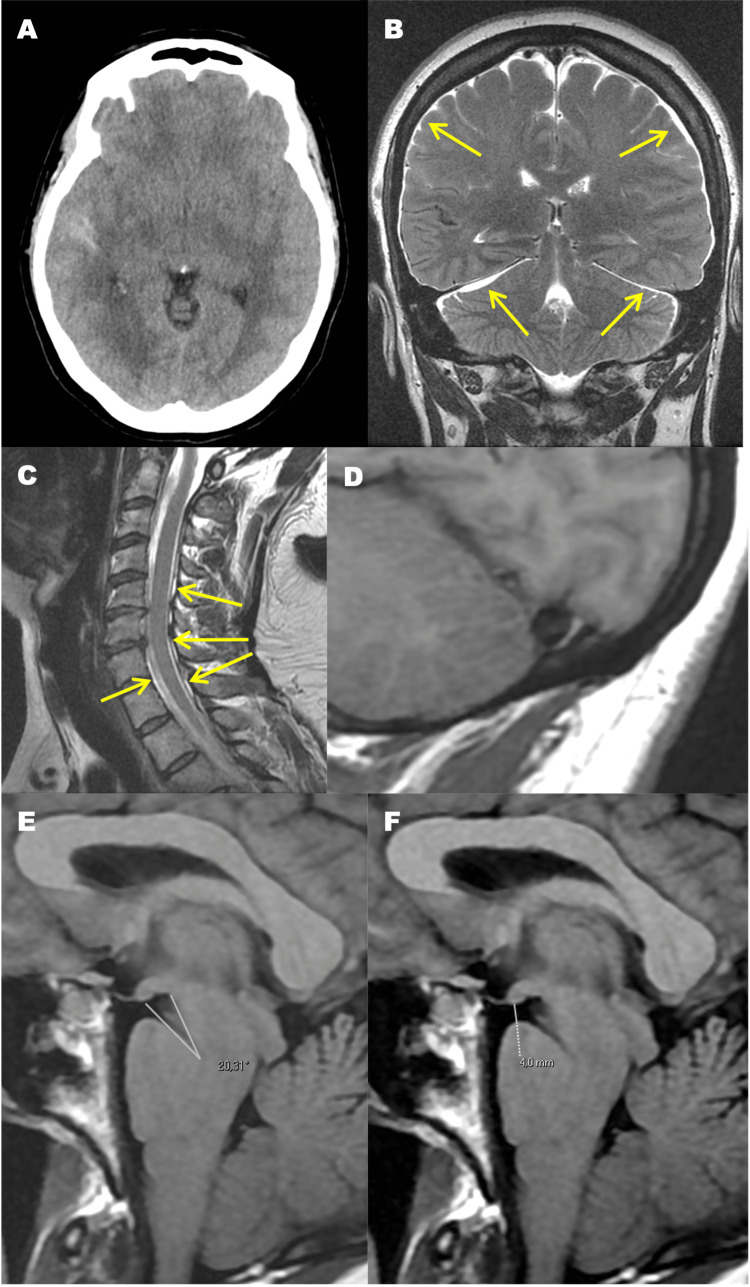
Imaging findings (A) CT brain scan, axial slice, showing subarachnoid hemorrhage on the right Sylvian fissure. (B) Brain MRI, coronal T2-weighted image, demonstrating subdural effusions (arrows). (C) Cervical MRI, sagittal T2-weighted image, showing spinal epidural collections (arrows). (D-F) Brain MRI, sagittal T1-weighted images, showing venous distension (D), a narrow pontomesencephalic angle (E), and a short mamillopontine distance (F).
